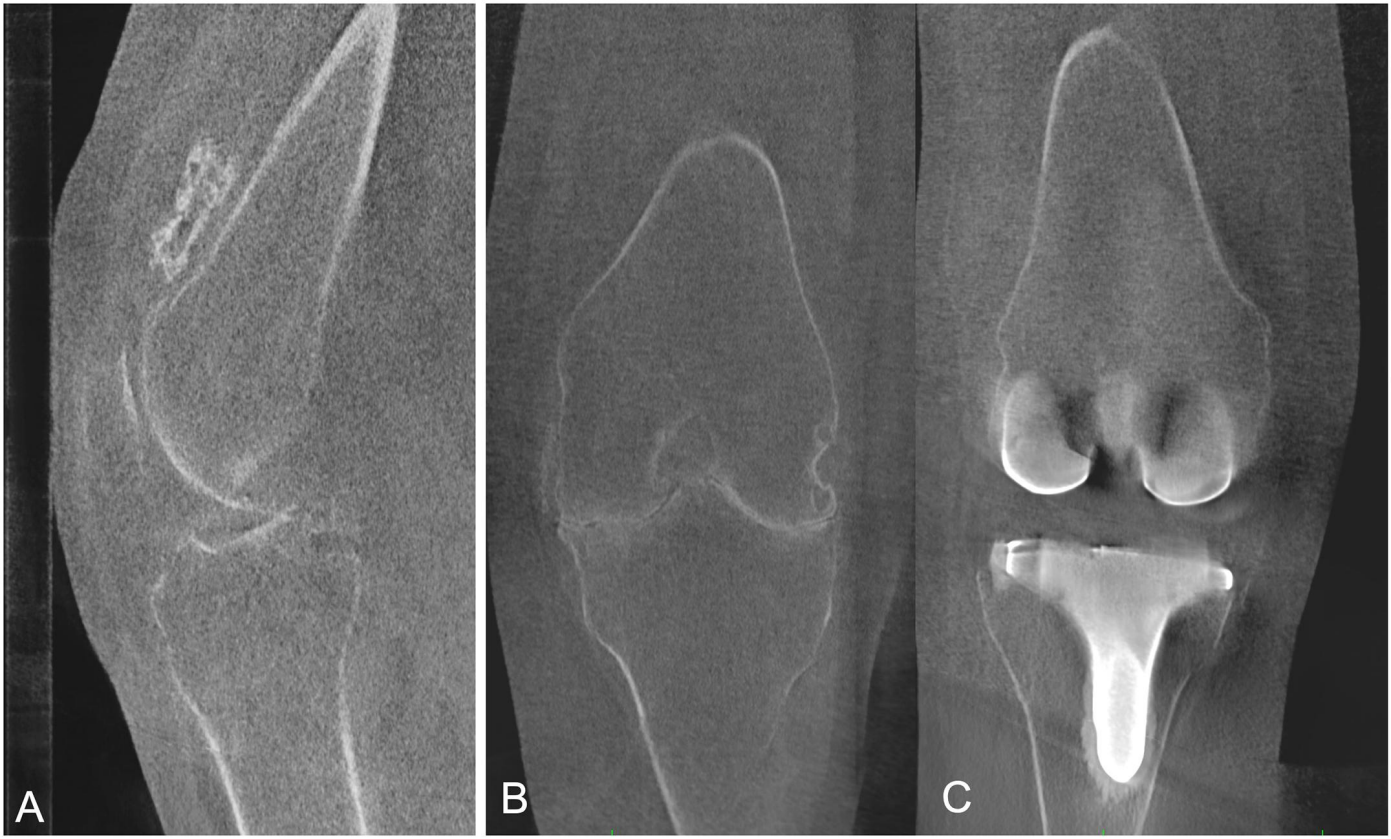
Weight-bearing CT in a patient with severe knee osteoarthritis. (A) Sagittal WBCT image shows joint bodies within the suprapatellar recess. (B) Coronal WBCT image demonstrates complete collapse of the medial joint space under load, consistent with advanced degenerative disease. (C) The contralateral knee arthroplasty is also visualized, without evidence of hardware complications.
